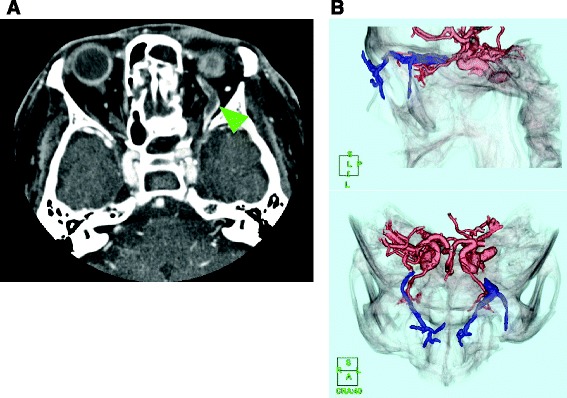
Contrast-enhanced computed tomography scan with angiography performed on day 12 to screen for superior ophthalmic vein engorgement. a The *yellow-green arrowhead* shows the dilated left superior ophthalmic vein (to 5.9 mm) together with a non-enhanced mass, which was indicative of thrombosis. b Computed tomography angiography did not detect any obvious arteriovenous fistulas or abnormal cavernous sinus enhancement
